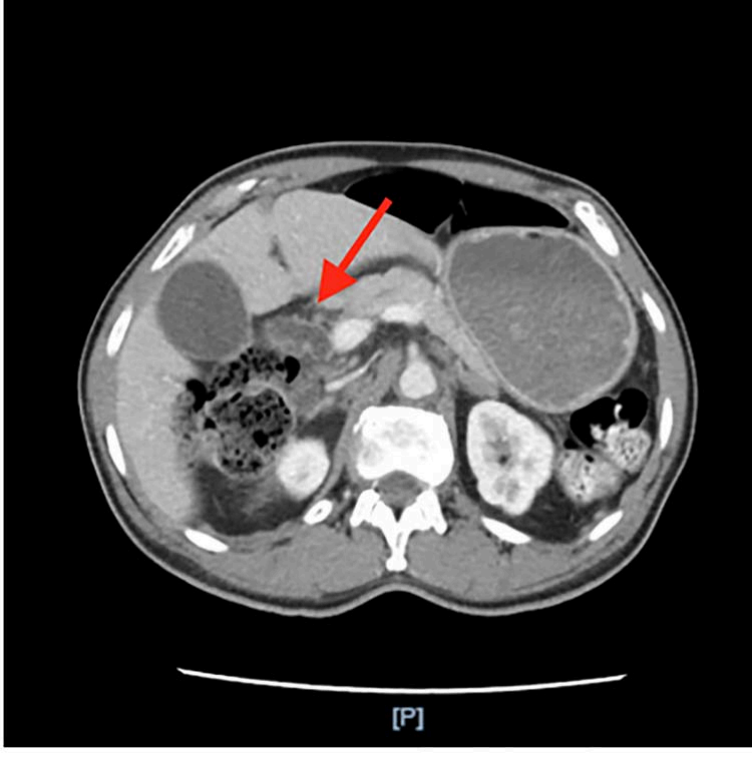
Dilated common bile duct on CT abdomen/pelvis.
